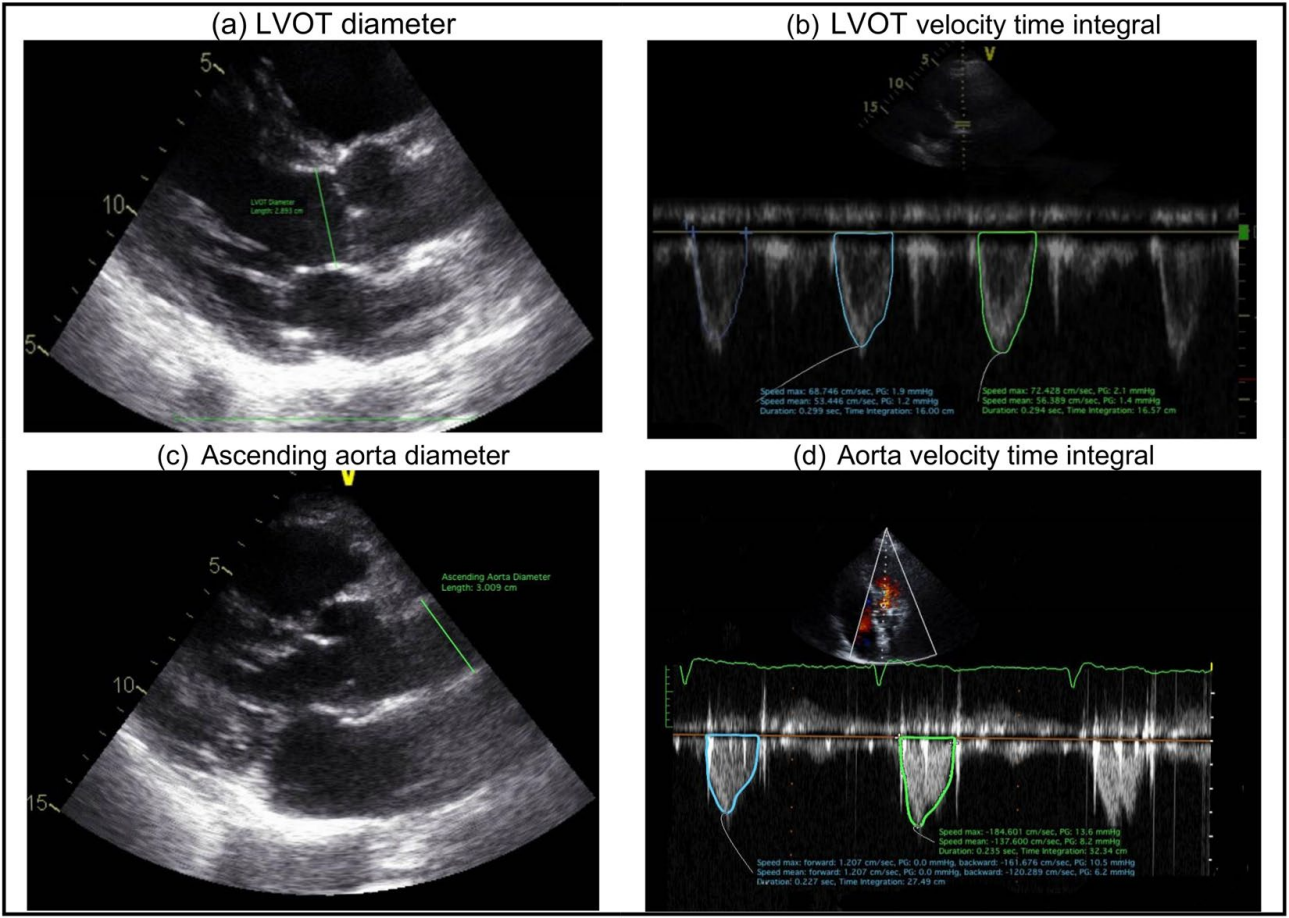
Doppler echocardiography measurements for left ventricular outflow tract and the aorta. (a) Left ventricular outflow tract diameter, measured in the parasternal long axis view; (b) left ventricular outflow tract velocity time integral, taken as the average of the areas; (c) Ascending aorta diameter, measured in the parasternal long axis view; (d) Aorta velocity time integral, taken as the average of the areas.
